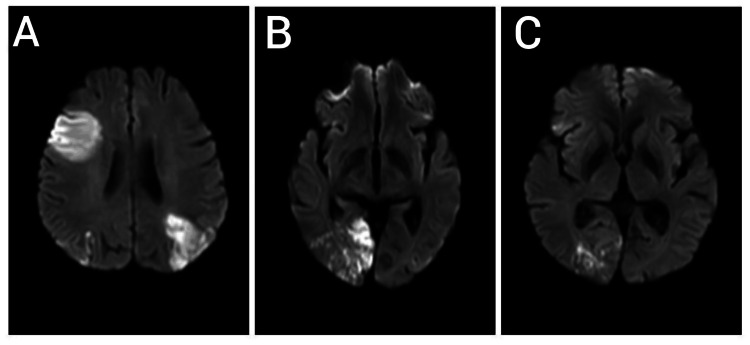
A, B, and C showing MRI brain suggestive of acute infarcts in the right frontal lobe, right insular cortex, bilateral parietal lobes, right posterior temporal lobes, and bilateral occipital lobes. MRI: magnetic resonance imaging
